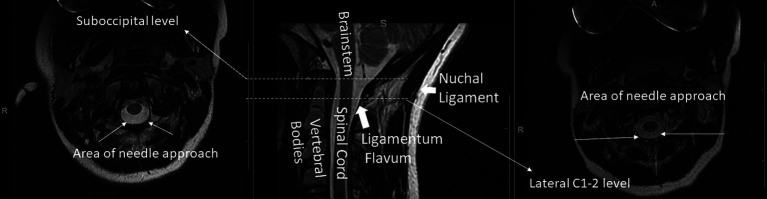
Axial T2 at the suboccipital (left) and lateral C1-2 (right) levels and corresponding mid-sagittal T2-weighted (middle) cervical spine MRI with a few key structures labeled.
